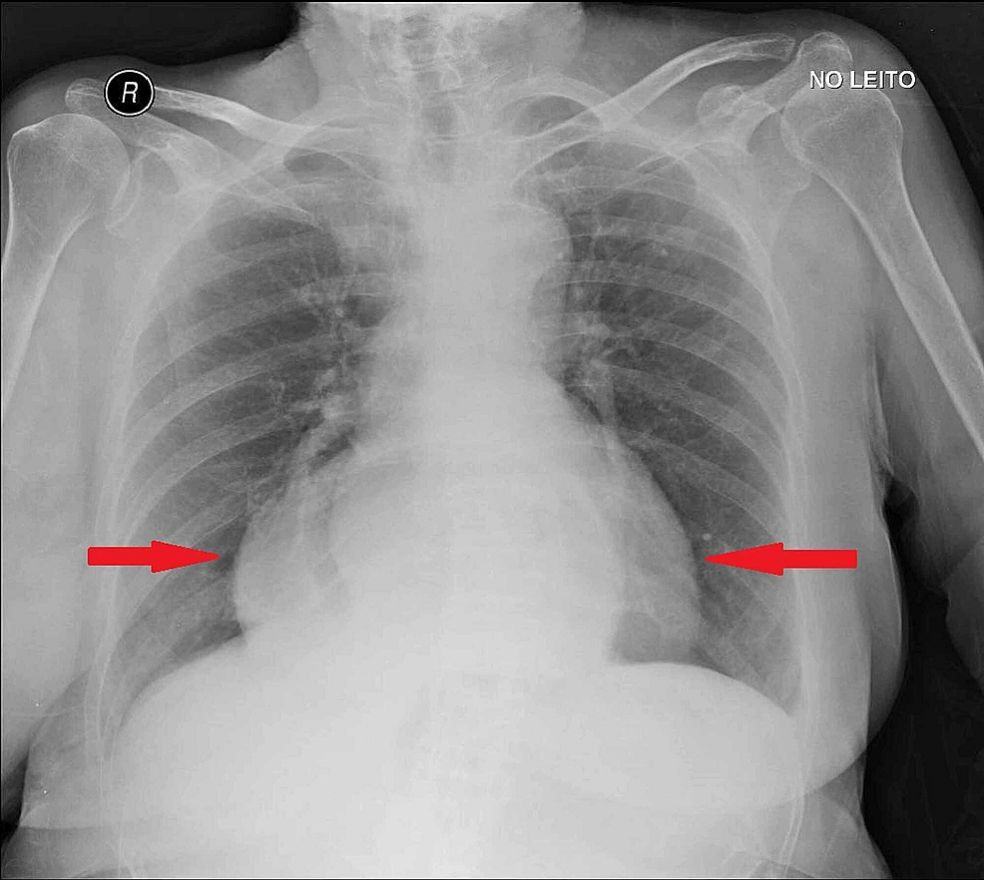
Chest radiography showing wide hernia of the hiatus May, 2020. The arrows show the stomach within the thoracic cavity.
